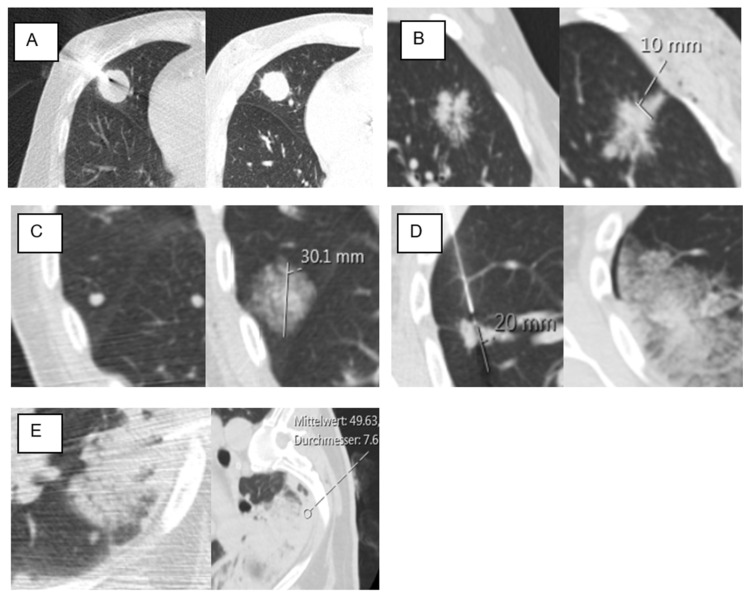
Pulmonary hemorrhage grading system on CT scans performed before/during (left) and directly after (right) the biopsy pulmonary hemorrhage. (A) Grade 0: without image morphological evidence of parenchymal hemorrhage after biopsy. (B) Grade 1: new focal hemorrhage less than 2 cm beyond the needle path can be delineated in the access route after the biopsy. (C) Grade 2: after the biopsy, the target lesion can no longer be demarcated if the alveolar hemorrhage is locally larger than 2 cm. (D) Grade 3: lobar hemorrhage after biopsy. (E) Grade 4: hemothorax with immediate hyperdense fluid within the pleural cavity after biopsy [23].
